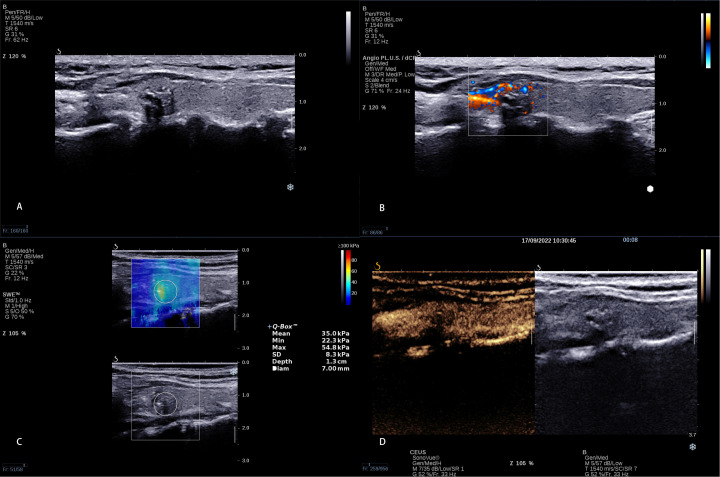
A 8 × 6 mm thyroid nodule in the left lobe of a 38-year-old woman. (A) Conventional ultrasound showed a solid hypo-echoic, irregular nodule with calcifications; the shape was wider than taller and the contact extent with adjacent thyroid capsule >25%. (B) AP shows that the vascularization of nodule could be classified as Grade I (C) SWE measurement shows that Emin, Emean, and Emax were 22.3 kPa, 35.0 kPa, and 54.8 kPa, respectively. (D) CEUS shows interrupted capsule at CEUS. The calculated predictive value with the logistic regression formula was 2.35 (>2.0805), which was regarded as presence of CLNM.
